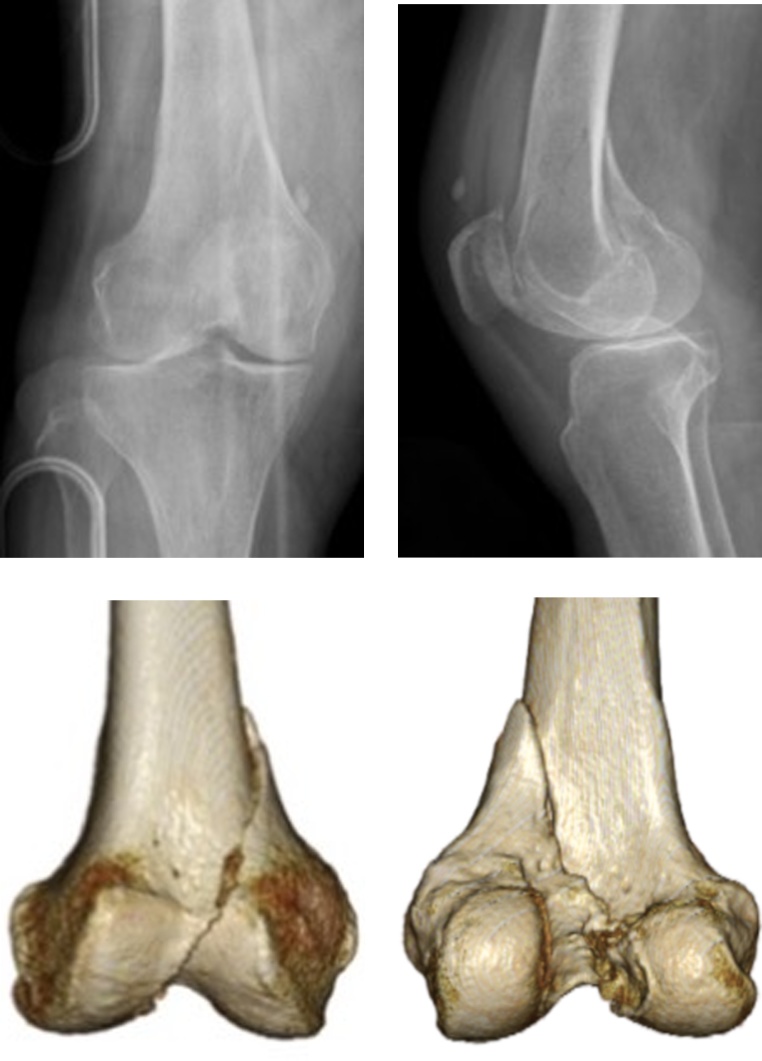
Plain radiography and computed tomography showed oblique fracture of the femoral medial condyle.
